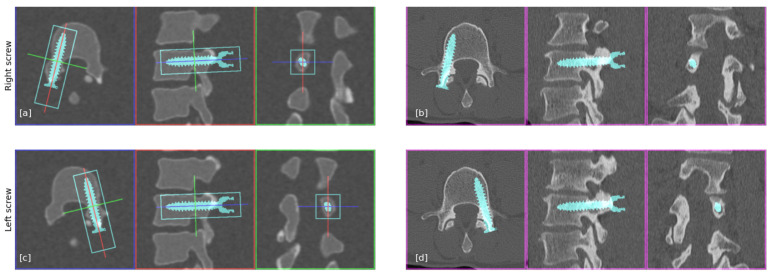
The planned screws on the sCT, and the way they will actually look in the real CT. Each sub-figure shows the screw placed in the 3 planes (axial, sagittal, and coronal). The colored lines are used to show the other planes according to the color. (a,c) Right/left screw as planned in the sCT. (b,d) Right/left screw as it will actually be placed in the CT.
